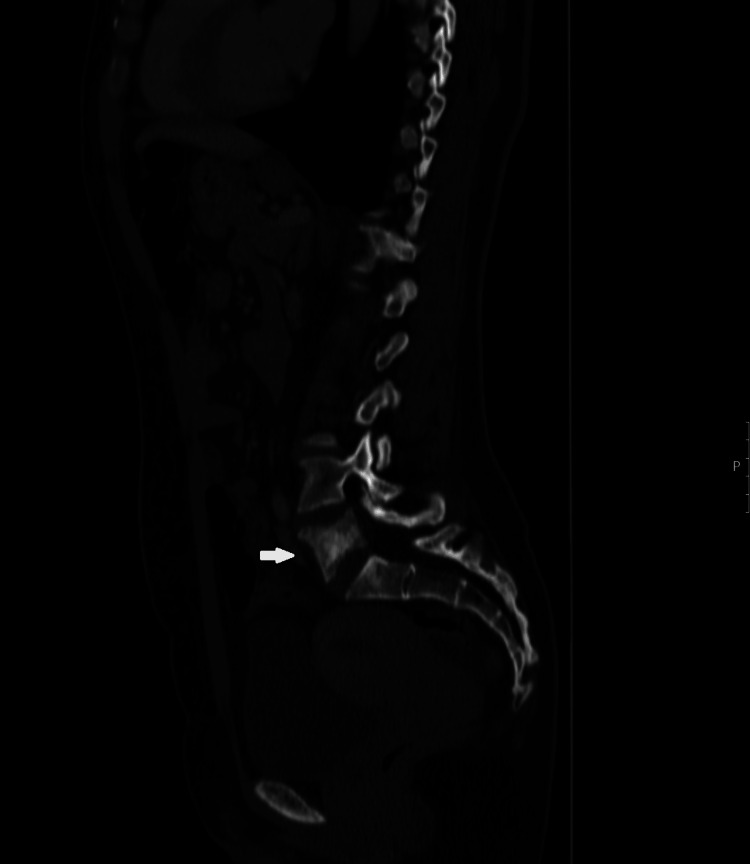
Lumbar spine CT scan, sagittal plane. A lumbar spine CT showed a lesion involving the body, left pedicle, and left superior articular facet of the L5 vertebrae.
